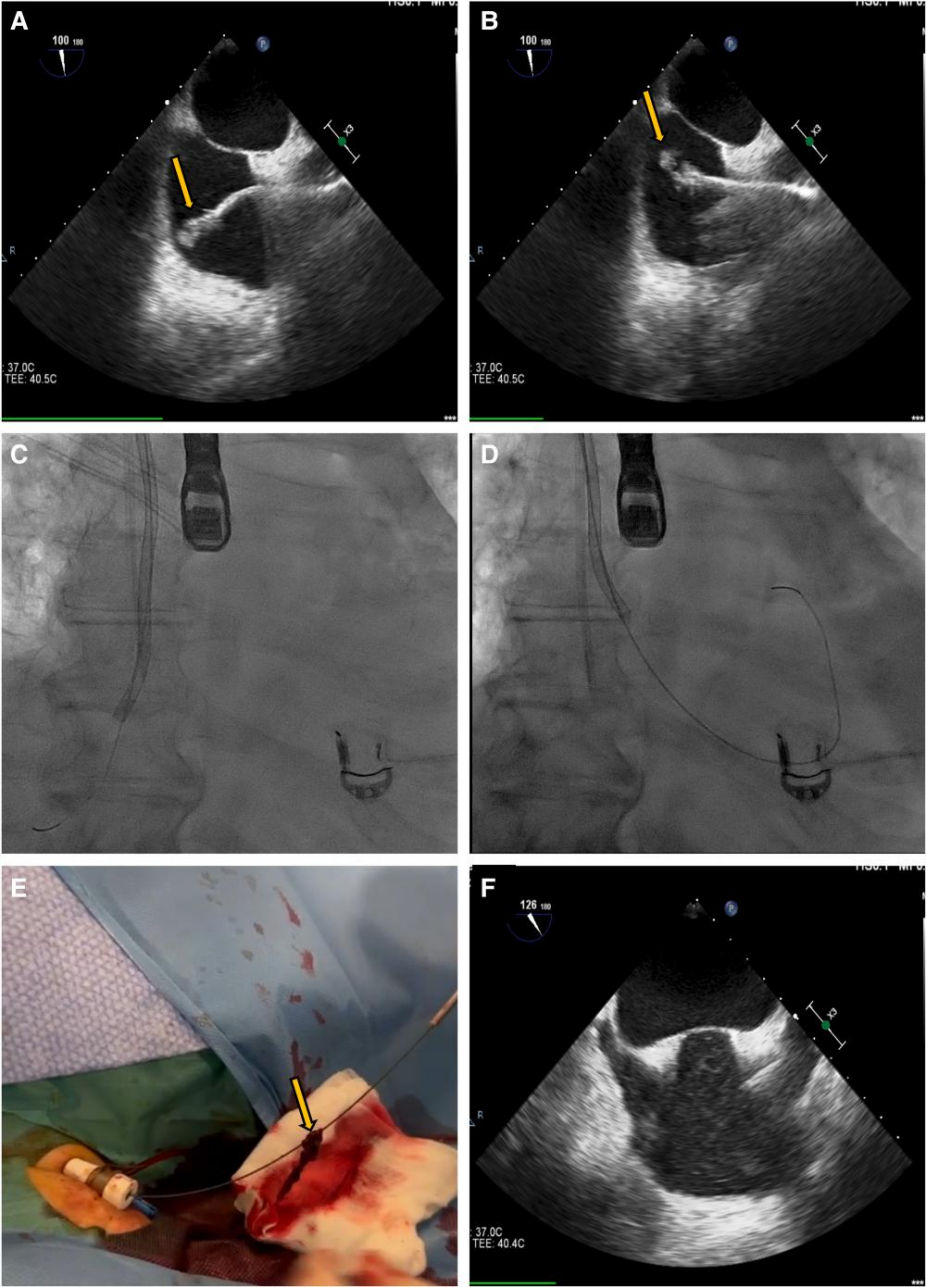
Intraprocedural characteristics. (*A* and *B*) Transoesophageal echocardiography-guided aspiration of the right atrial thrombus using the Penumbra Indigo Lightning System (arrow). (*C* and *D*) Fluoroscopic guidance of thrombus aspiration with the Penumbra catheter advanced over a 0.032′ Storq guidewire used as a separator. (*E*) The extracted thrombotic material (arrow). (*F*) The complete removal of the right atrial thrombus following catheter-directed therapy, as demonstrated by the absence of residual thrombus on echocardiography.
